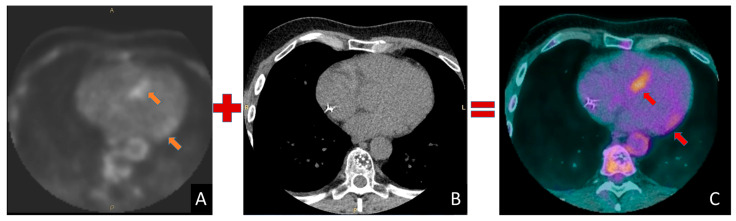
Representative example of a PET-TC merged image. (A) PET shows increased FDG uptake in the basal septum and basal lateral wall of the left ventricle (arrows). (B) chest CT image, (C) merged PET and TC image that allows better localization of the areas with increased uptake (arrows).
